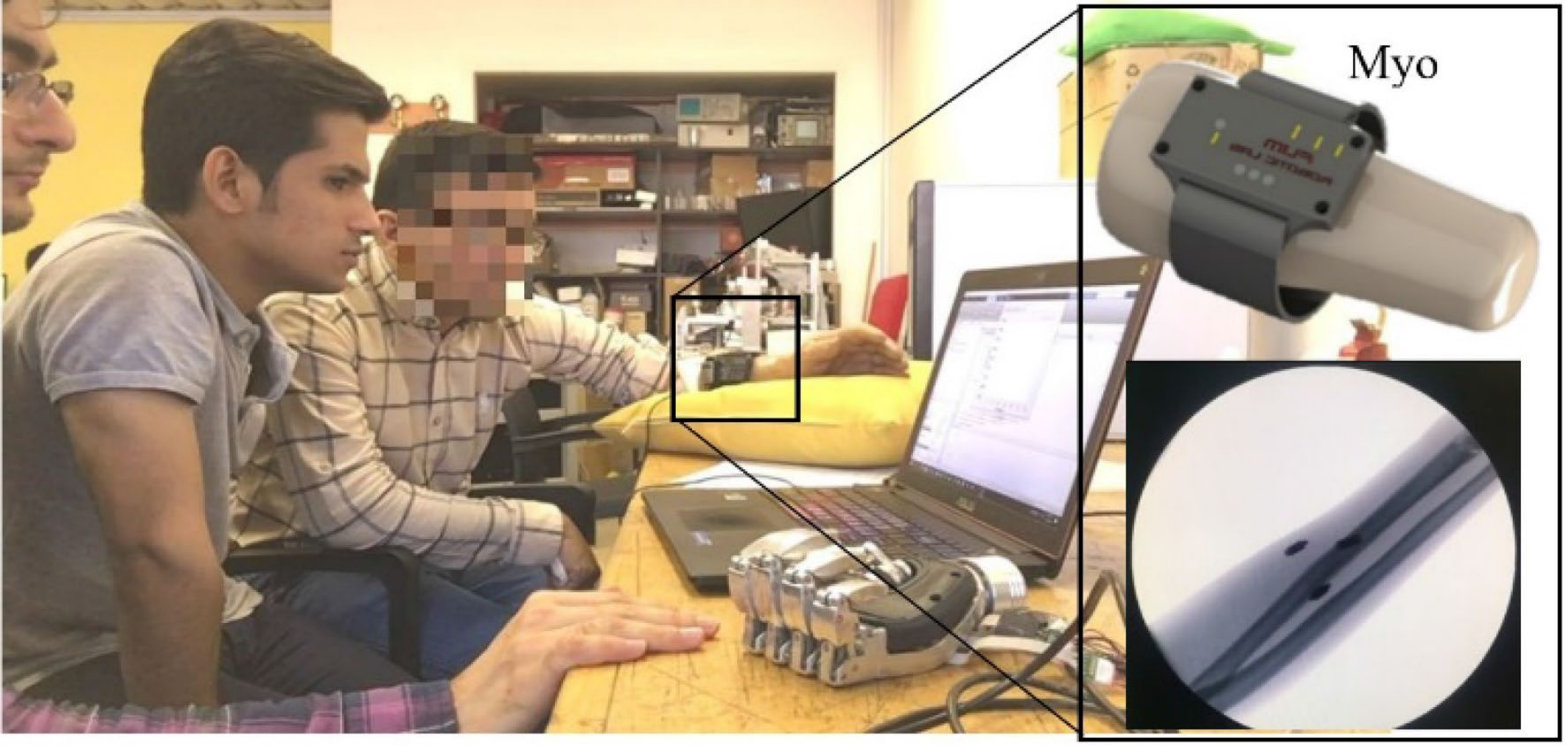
Connection of the prosthesis to the Myo along with an x-ray that shows the placement of magnets inside the patient's amputated arm. The measured magnetic fields by the Myo sensors are immediately transferred to a computer. Processing the measured signals is carried out by MATLAB software to map the magnetic field to the bionic hand movement. These movements are then fed to the present bionic hand motors for the appropriate gesture. In this picture, the patient has voluntarily closed his prosthesis hand. The participants gave written consent to include their images in this article.
